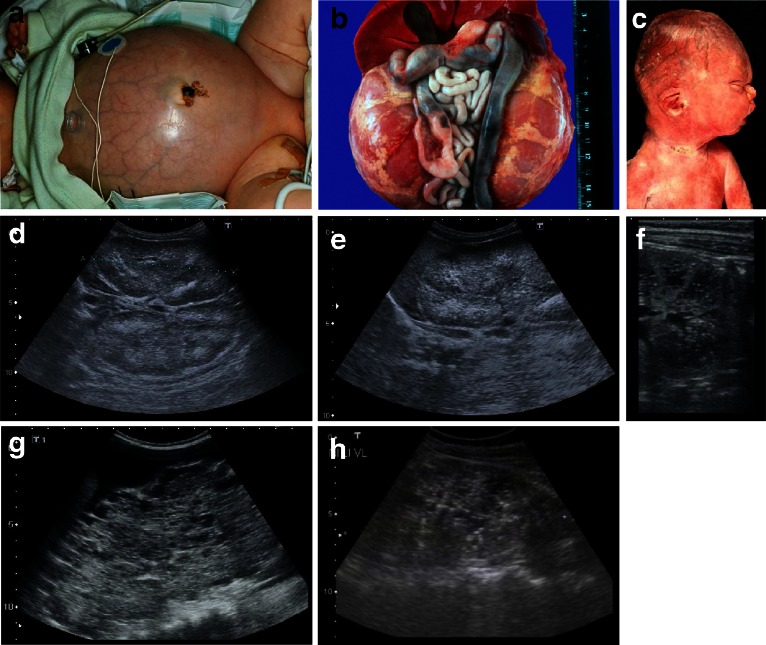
a–h Autosomal recessive polycystic kidney disease (ARPKD). a Baby with distended abdomen due to voluminous kidneys that led to respiratory problems and early demise. b Abdominal situs of a perinatally demised ARPKD patient with symmetrically enlarged kidneys that maintained their reniform configuration. c Potter’s phenotype with distinctive facial features due to a lack of amniotic fluid. d–h Renal ultrasound scans of babies and young children with ARPKD. Symmetrically enlarged echogenic kidneys with fusiform dilations of collecting ducts and distal tubules are arranged radially throughout the renal parenchyma from medulla to cortex
